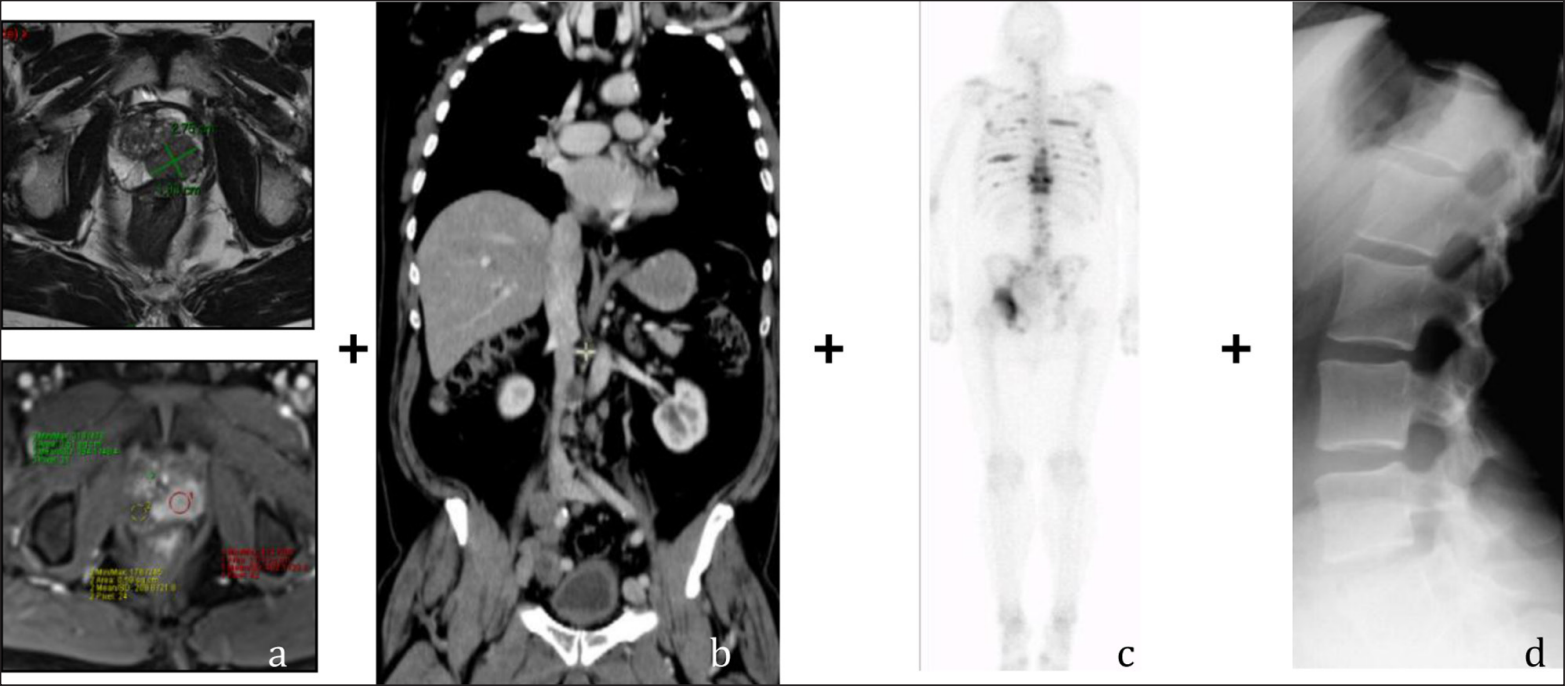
The multiparametric protocol (a) mp-MRI for the T staging (b) Thoraco-abdominal CT for the N staging (c) BS (d) Targeted X-Rays for the M-Staging.
